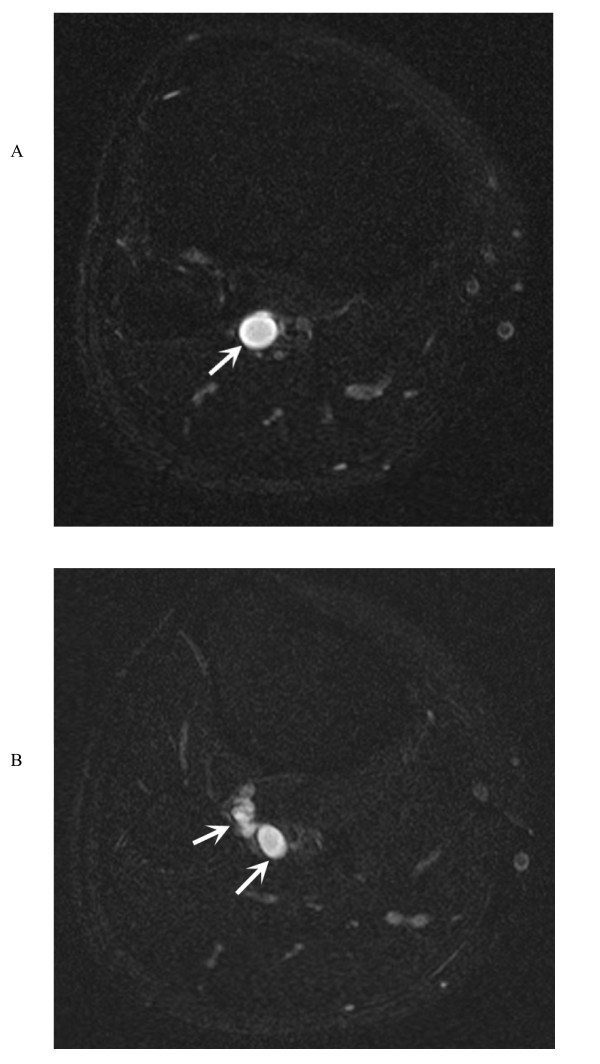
Axial T2-weighted fat saturated turbo spin echo images demonstrate a well defined, homogeneously hyper-intense multi-lobulated cystic lesion in the right popliteal fossa (Figure 1a and 1b, arrows).
